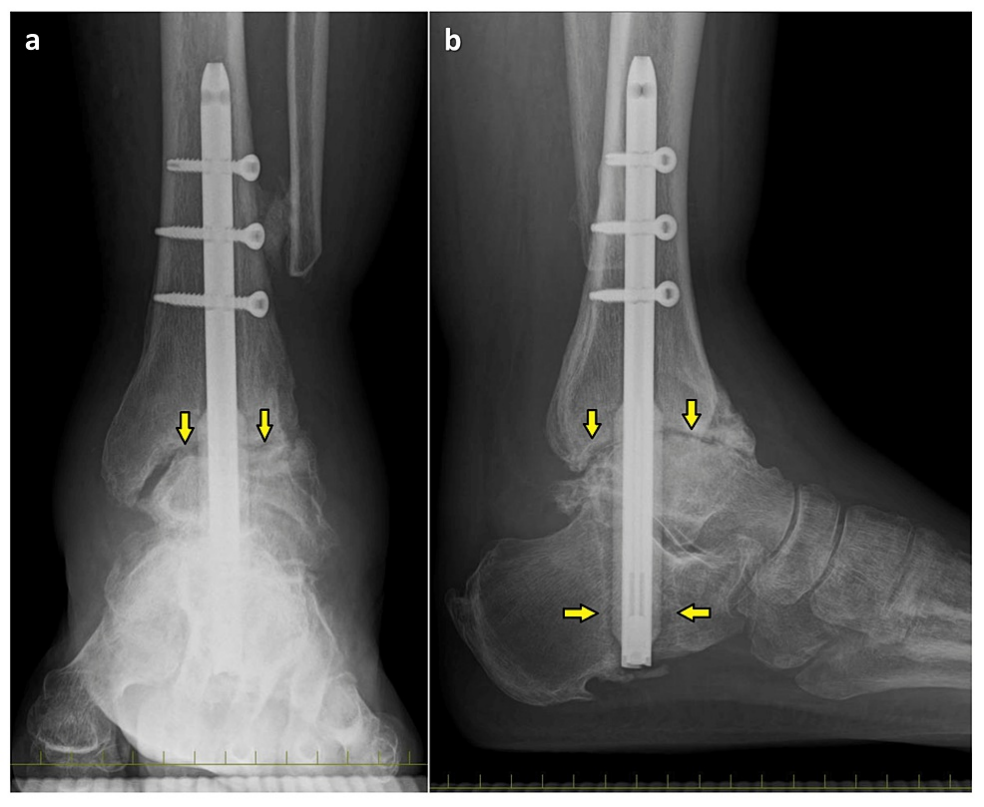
Preoperative plain radiographs at standing position (a) anteroposterior and (b) lateral images
